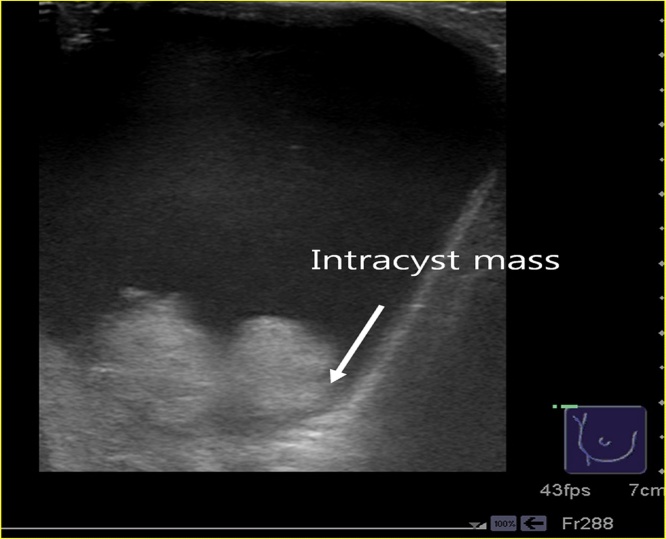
Ultrasonographic finding of the cyst. There is a 2 × 2.5 cm sized hyper-echoic, polypoid mass (arrow) in the huge cyst.
